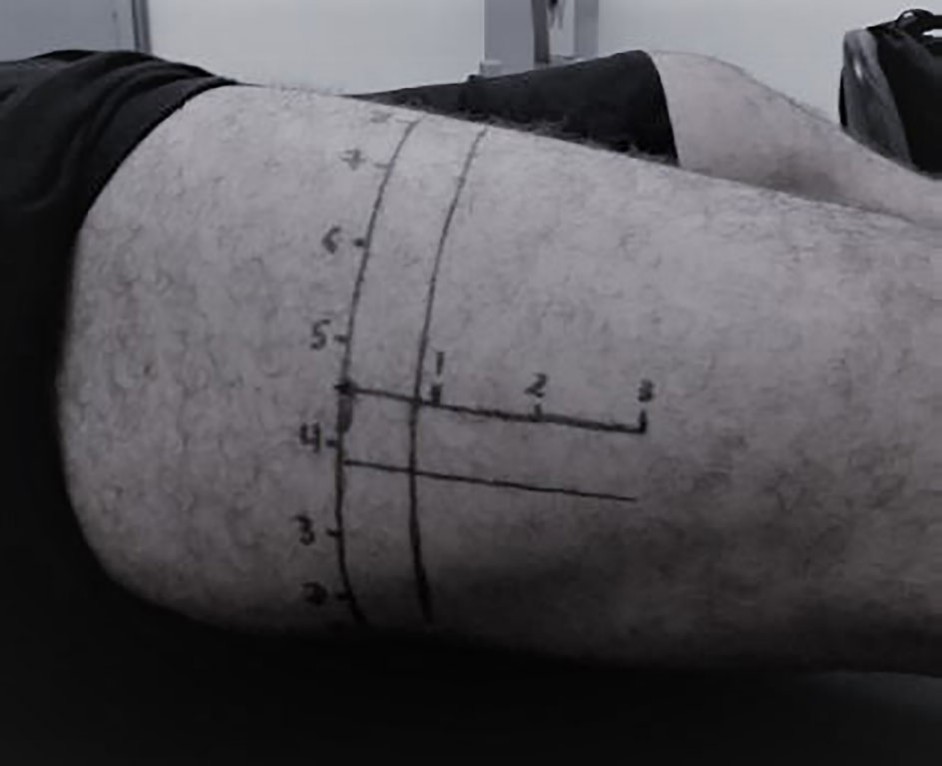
Marking template for ultrasound assessment.
